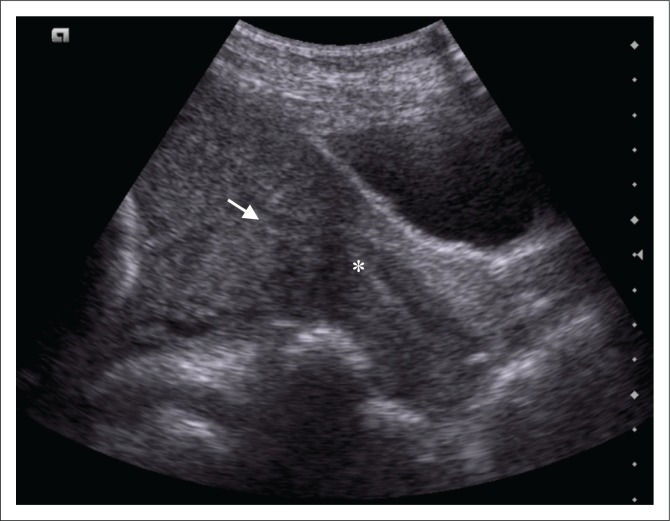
Longitudinal abdominal ultrasound demonstrating placental tissue (white arrow) overlying the internal ostium (os) of the cervix (asterisk).
